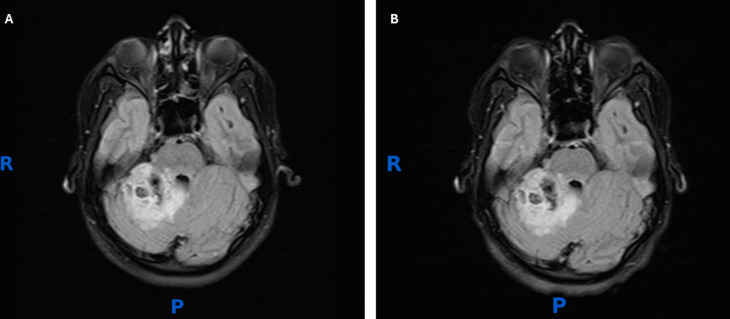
Serial non-contrast axial T2-weighted magnetic resonance images (MRI) of the posterior fossa lesion. A Initial MRI showing a well-circumscribed heterogeneous, solid cystic lesion (44 × 44 mm) centered on the right middle cerebellar peduncle and superior cerebellar vermis, with associated mass effect and partial effacement of the fourth ventricle; and no hydrocephalus. The lesion is hyperintense. B Follow-up MRI demonstrating interval stability of the right cerebellar lesion without evidence of significant interval change in size (45 × 39 × 36 mm) or characteristics, consistent with an indolent lesion. Susceptibility-weighted imaging (SWI) sequences (not shown) demonstrated a blooming artifact consistent with altered blood products within the center of the lesion
